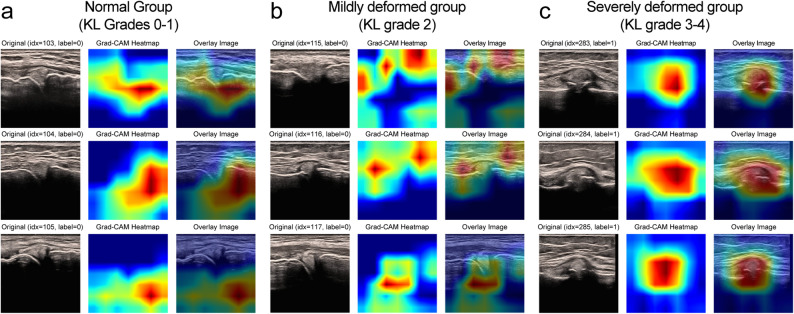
Representative Grad-CAM activation regions by KL grade. (A) Representative Grad-CAM heat maps for OA diagnosis in the non-OA group (KL grades 0–1). (B) Representative Grad-CAM heat maps for OA diagnosis in the mild OA group (KL grade 2). (C) Representative Grad-CAM heat maps for OA severity classification in the severe OA group (KL grades 3–4). From left to right, the panels show the original ultrasound image, the Grad-CAM heat map, and the heat map overlaid on the original image
